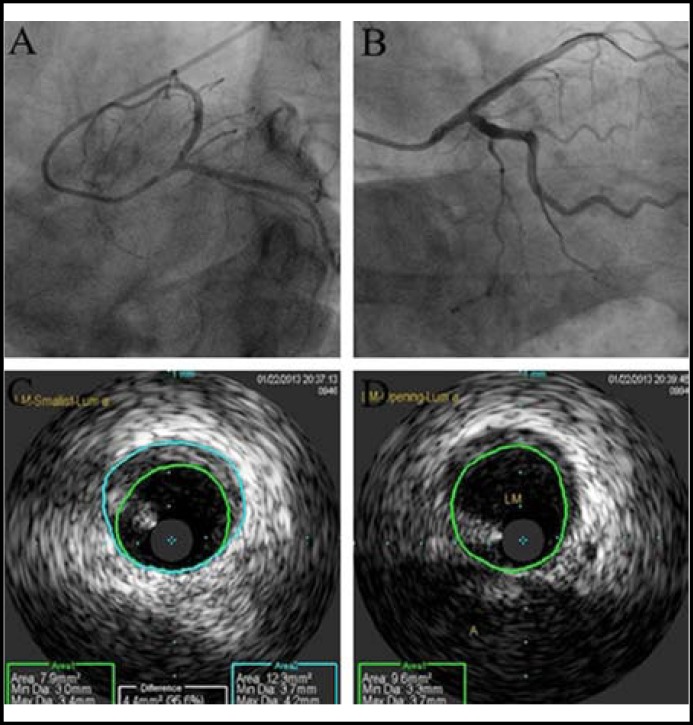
Coronary angiography showing diffuse left main coronary artery vasospasm (A) and resolution of the spasm after administration of intracoronary nitrates (B). Intravascular ultrasound showing a minimal concentric plaque in the left main coronary artery with minimum lumen area of 7.9mm^2^(C) and with maximum lumen area of 9.6mm^2^(D).
